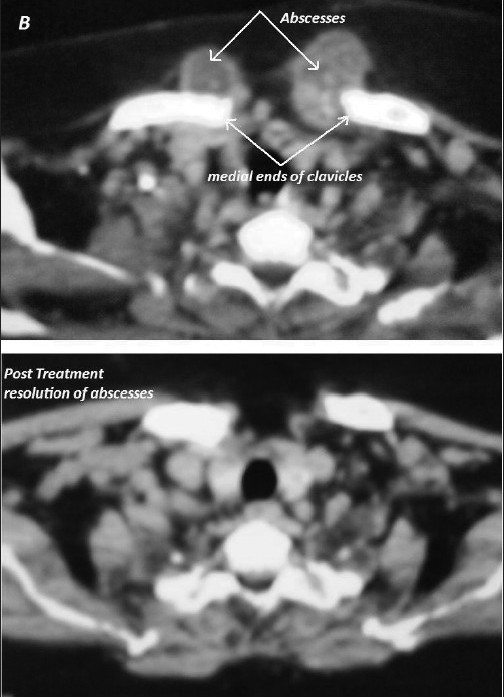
Computed tomography (CT) scan showing large fluid collections centred around bilateral sternoclavicular joints with erosions involving medial ends of clavicles and sternum (upper) and Follow up CT scan showing complete resolution of abscesses after antitubercular therapy (lower)
